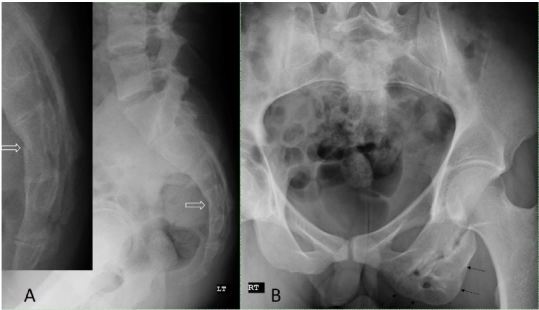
(A) Lateral pelvic radiograph demonstrates a minimally displaced transverse fracture of the S4 vertebrae (open arrows); (B) Anteroposterior pelvic radiograph reveals an incidental old left ischial apophysial avulsion injury (arrows).
